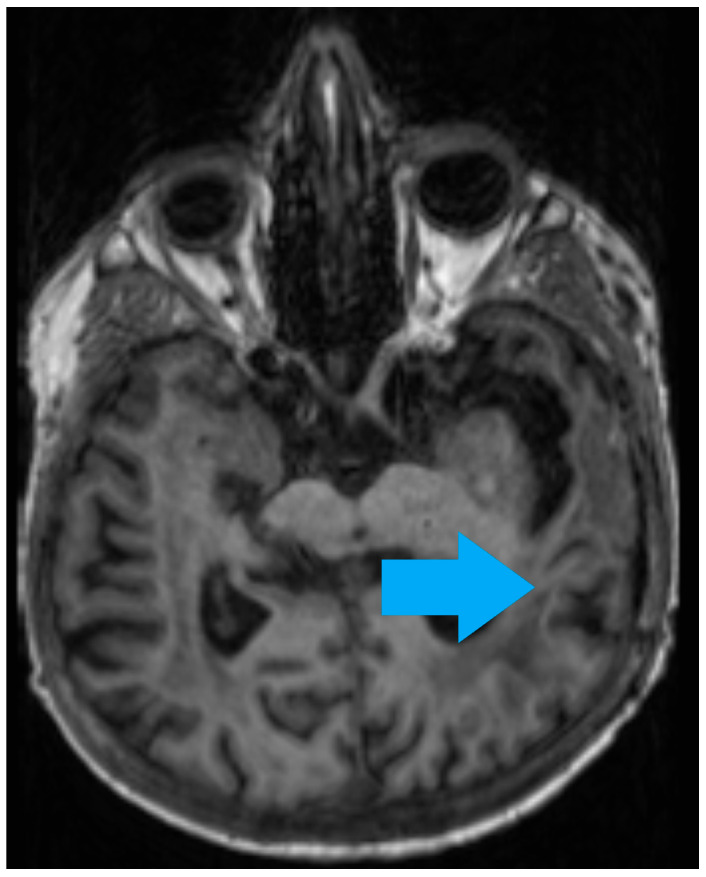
Brain MRI—partial regression of the lesions.
